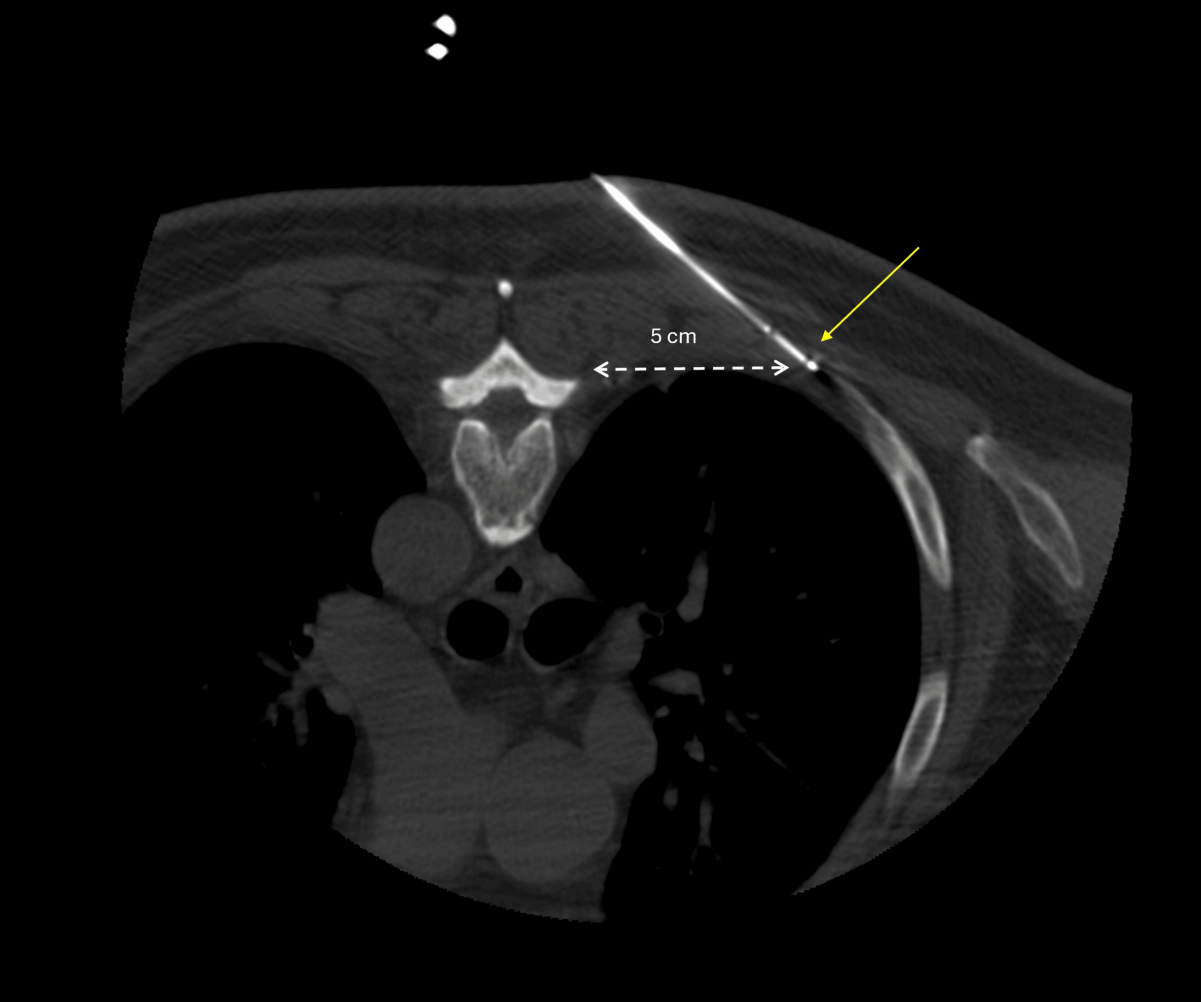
Intraprocedural axial computed tomographic image of the cryoablation probe placement (yellow arrow), at the level of the affected rib with ablation zone distancing 5 mm away from the subcostal groove and 5 cm away from the thoracic nerve roots
